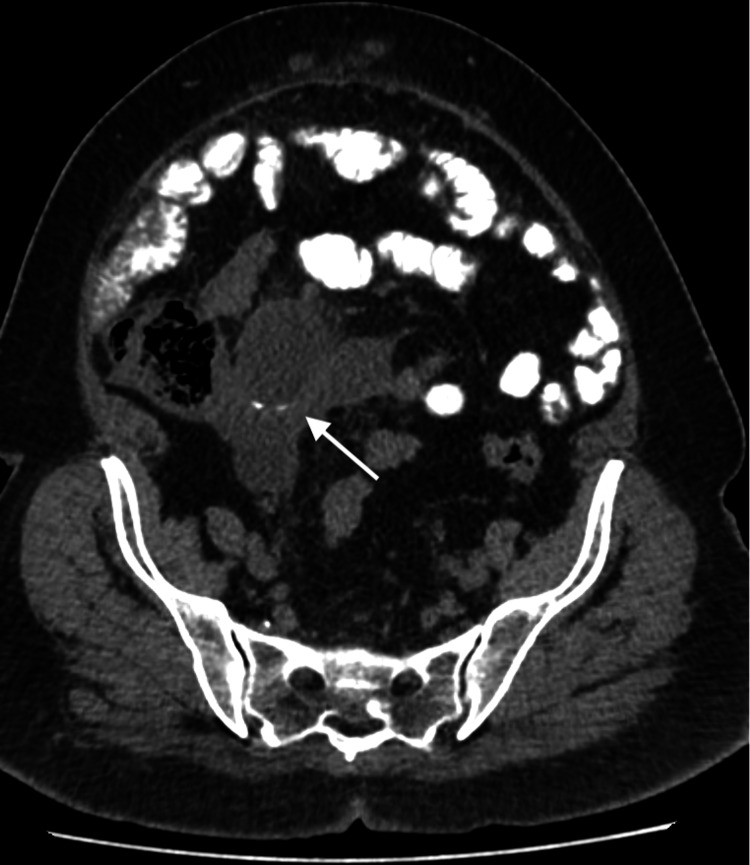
Axial CT imaging of the lower abdomen with administration of oral contrast. Axial view of the lower abdomen demonstrates a large irregular hypodense mass in the right lower quadrant inseparable from the cecal pole. A rounded, well circumscribed cyst is seen within the mass and is associated with a curvilinear calcification on its wall.
